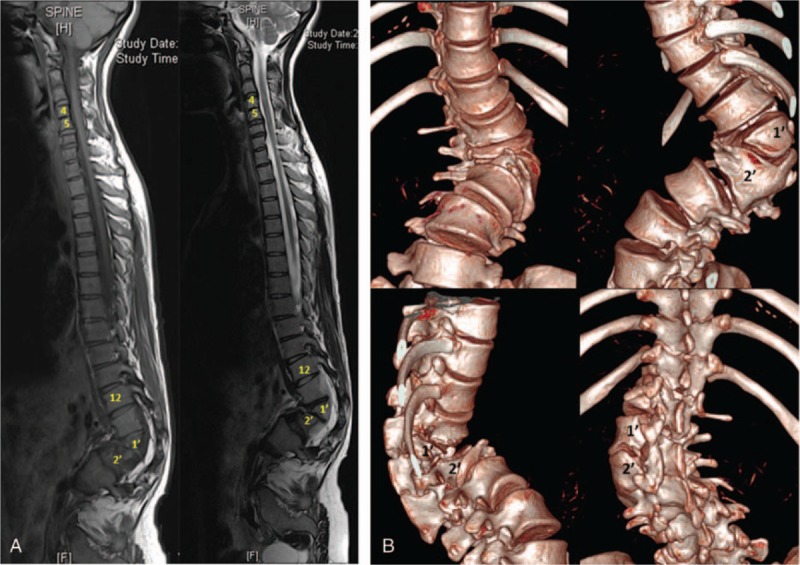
1–2 A thoracolumbar 3-dimensional computed tomography reconstruction and spinal magnetic resonance imaging revealed dysplasia of the first and second lumbar vertebral bodies (hemivertebra) and of the fourth and fifth cervical vertebral bodies (failure of segmentation), in addition to thoracolumbar kyphoscoliosis.
